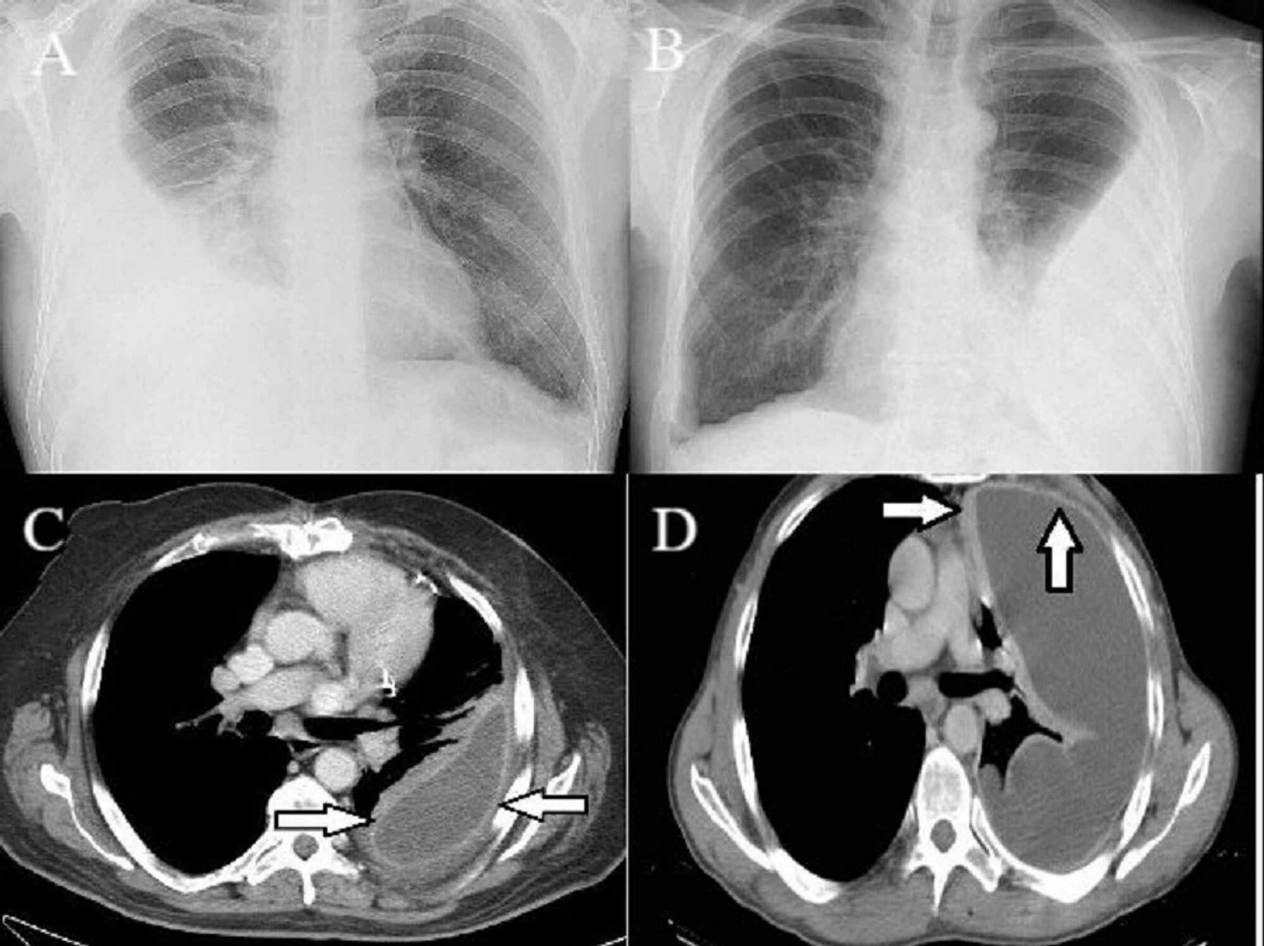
(A) Radiographic findings of empyema thoracis; (B) X-ray of empyema thoracis; (C) and (D) split pleura sign depicting chronicity marked with arrows on CT
